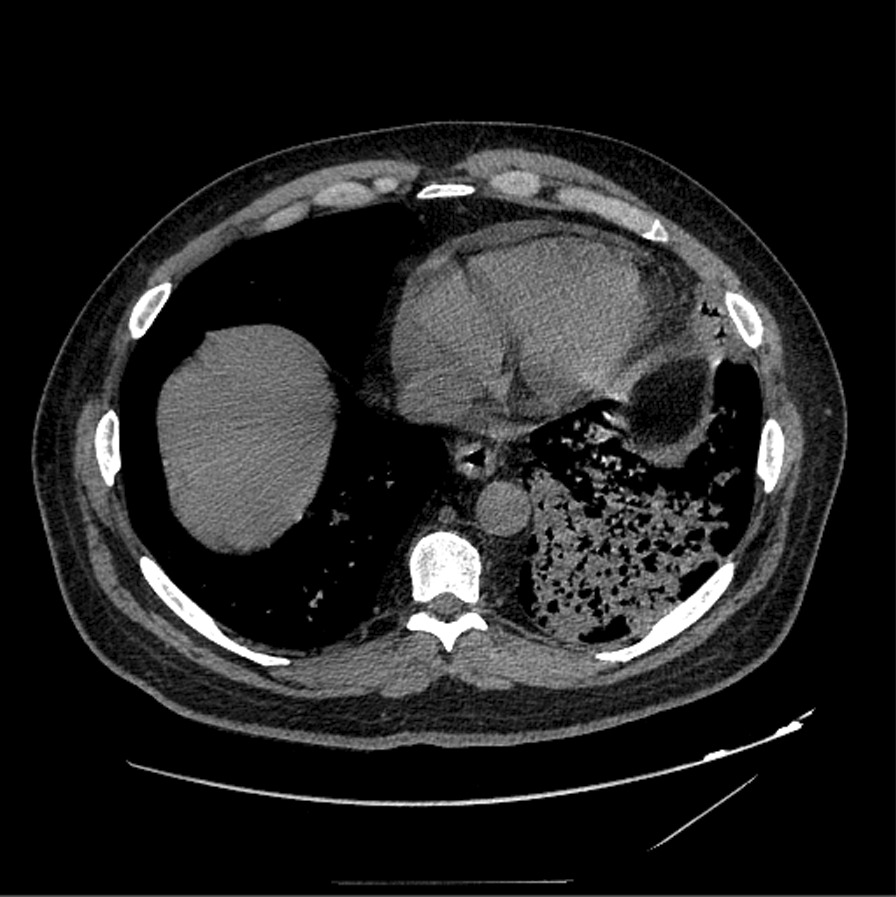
44 year old male with acute coccidioidomycosis presenting with fever, cough, and shortness of breath. Axial CT image at the level of the lower chest demonstrates a small pericardial effusion with extensive consolidation at the left lung base
